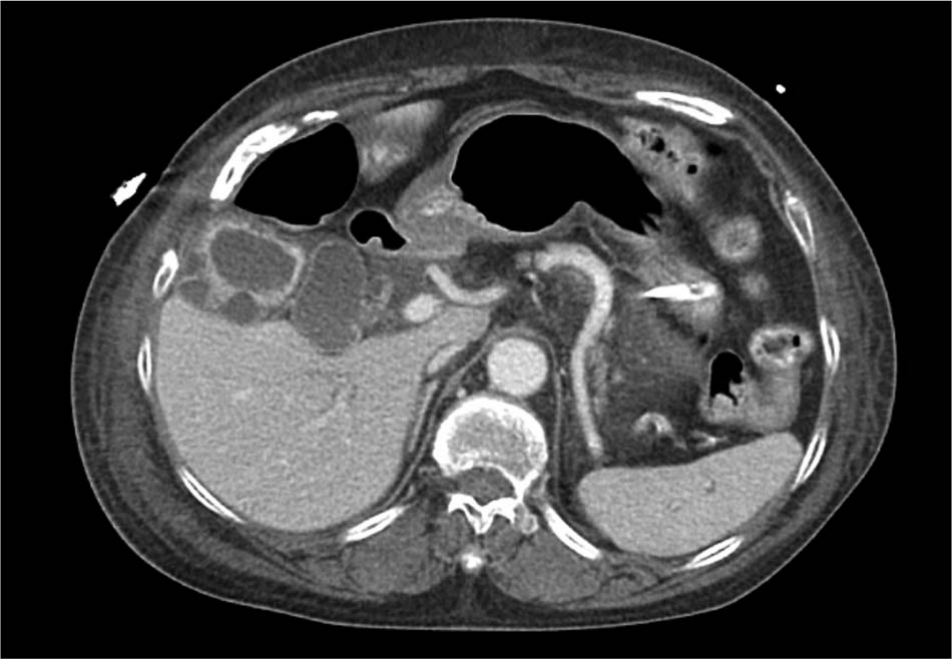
CT chest abdomen pelvis with IV contrast axial view. Gallbladder wall hyperenhancement and multiple intramural hyperattenuating nodes are again apparent in axial imaging.
